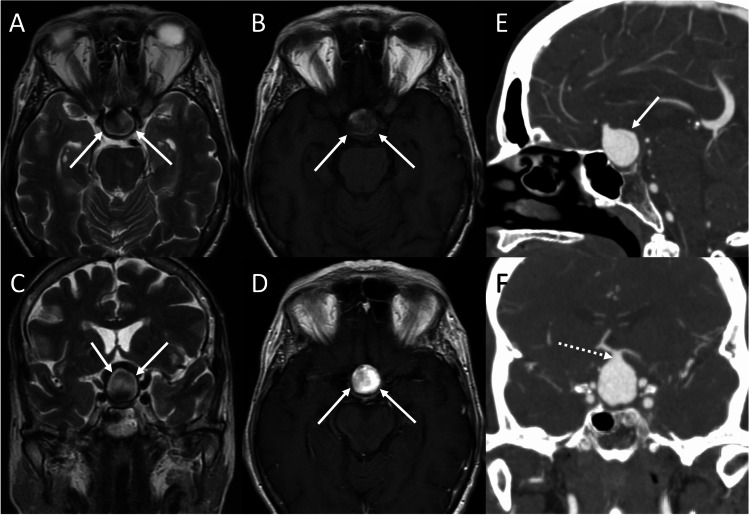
Anterior communicating artery aneurysm. Axial T2- (A) and T1- (B), coronal T2- (C), and axial contrast-enhanced T1-weighted (D) MR images display an intra- and suprasellar round expansile lesion (arrows) featuring inhomogeneous T1- and T2-signal due to flow artifact and presenting homogeneous enhancement. Sagittal (E) and coronal (F) CT angiography reformats confirm the presence of a saccular aneurysm whose neck is located at the level of the anterior communicating artery (dotted arrow)
